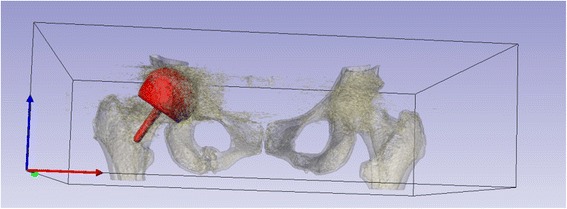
3D CT methodology. An example of the co-registration process within the software used, showing the *in vivo* position of the implant within the pelvis
